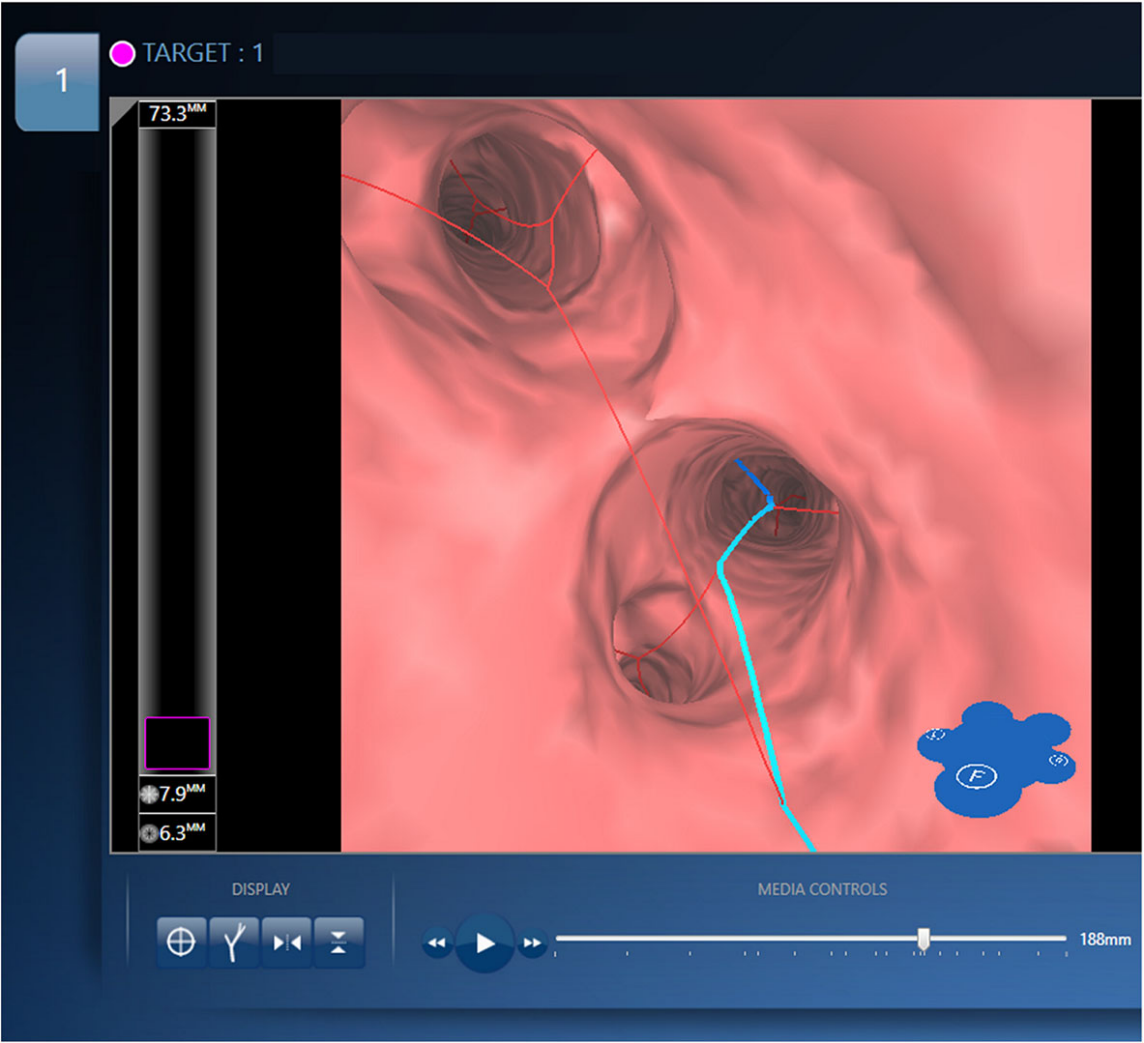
Guidance screen of the VBN. The red line represents all bronchial pathways and the blue line is the automatically searched-for route with the closest access to the virtual target. The navigation direction accuracy was defined as the concordance in the progression of the blue line between the VBN using low dose CT and that using standard dose CT. The number of branching’s was defined as the number of red lines that directly branched from the blue line, and the accuracy of the number of branching’s was defined according to the concordance of this number between the VBNs using low dose and standard dose CT. VBN = virtual bronchoscopy navigation
